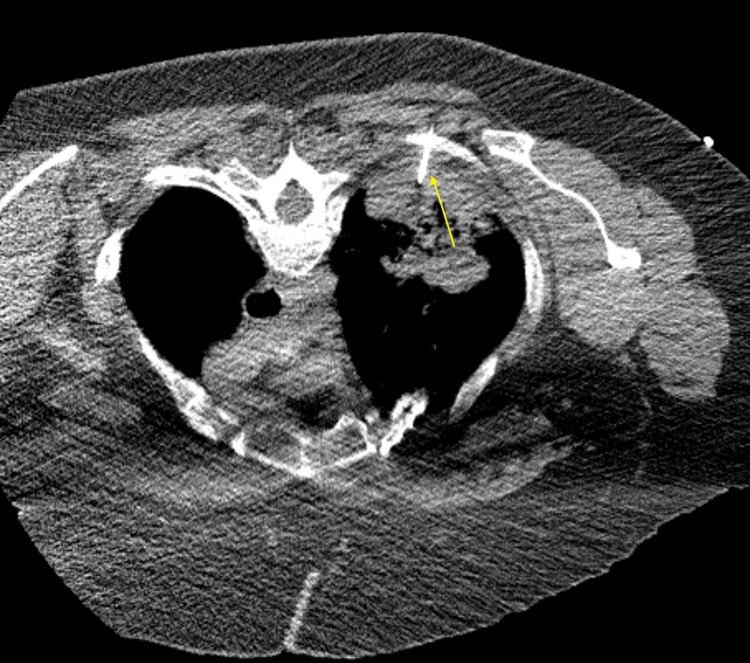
Demonstration of repeated CT-guided biopsy of a left upper lobe lung lesion, as indicated by a yellow arrow CT: computed tomography
